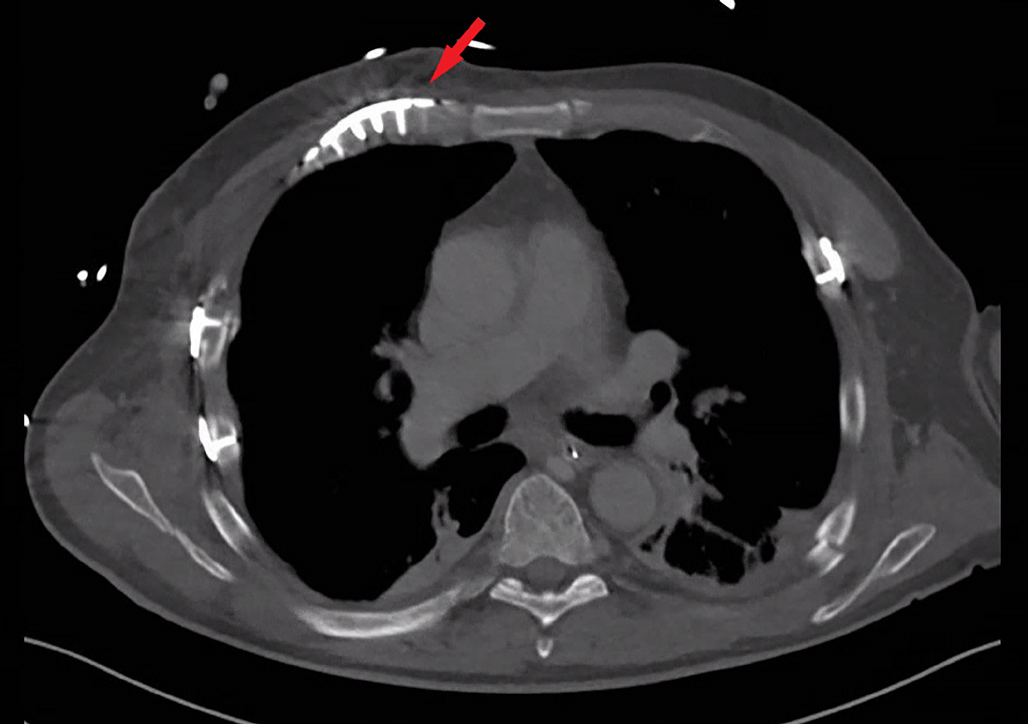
CT scan showing the fixated costochondral defect of the right third rib at 7 months after trauma (arrow).
